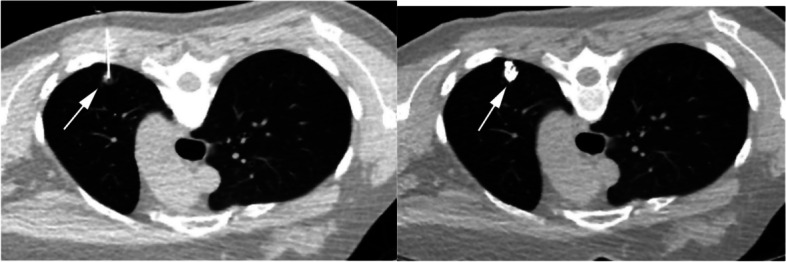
The CT-guided lipiodol localization [10]
